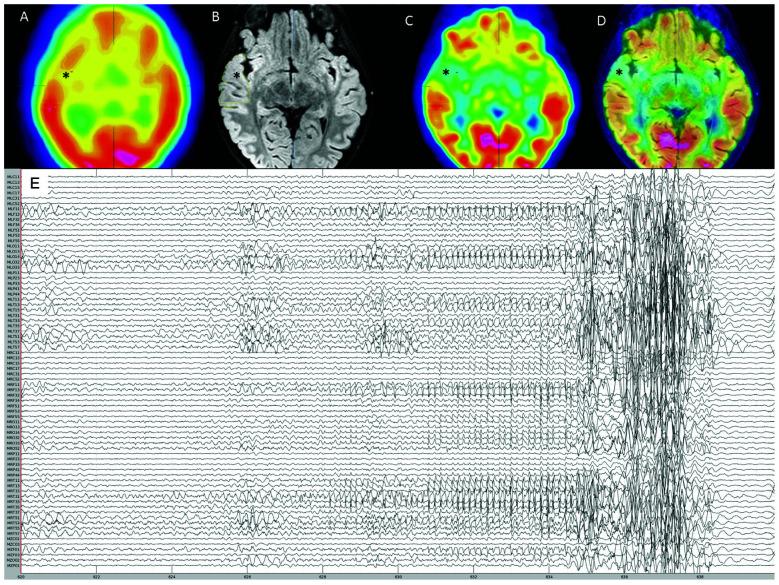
Pre-surgical evaluation neuroimaging. (A) Interictal SPECT showing right anterior temporal hypoperfusion (*). (B) 3T-magnetic resonance imaging (MRI) FLAIR sequence showing right anterior thickened cortex and blurring of gray matter-white matter junction (*). (C) FDG-PET showing right anterior temporal hypometabolism (*). (D) Co-registration of 3T-MRI FLAIR sequence with FDG-PET showing concordance between these imaging modalities (*). (E) Reduced MEG montage (76/275 MEG channels displayed) showing the seizure onset followed by movement artifact.
